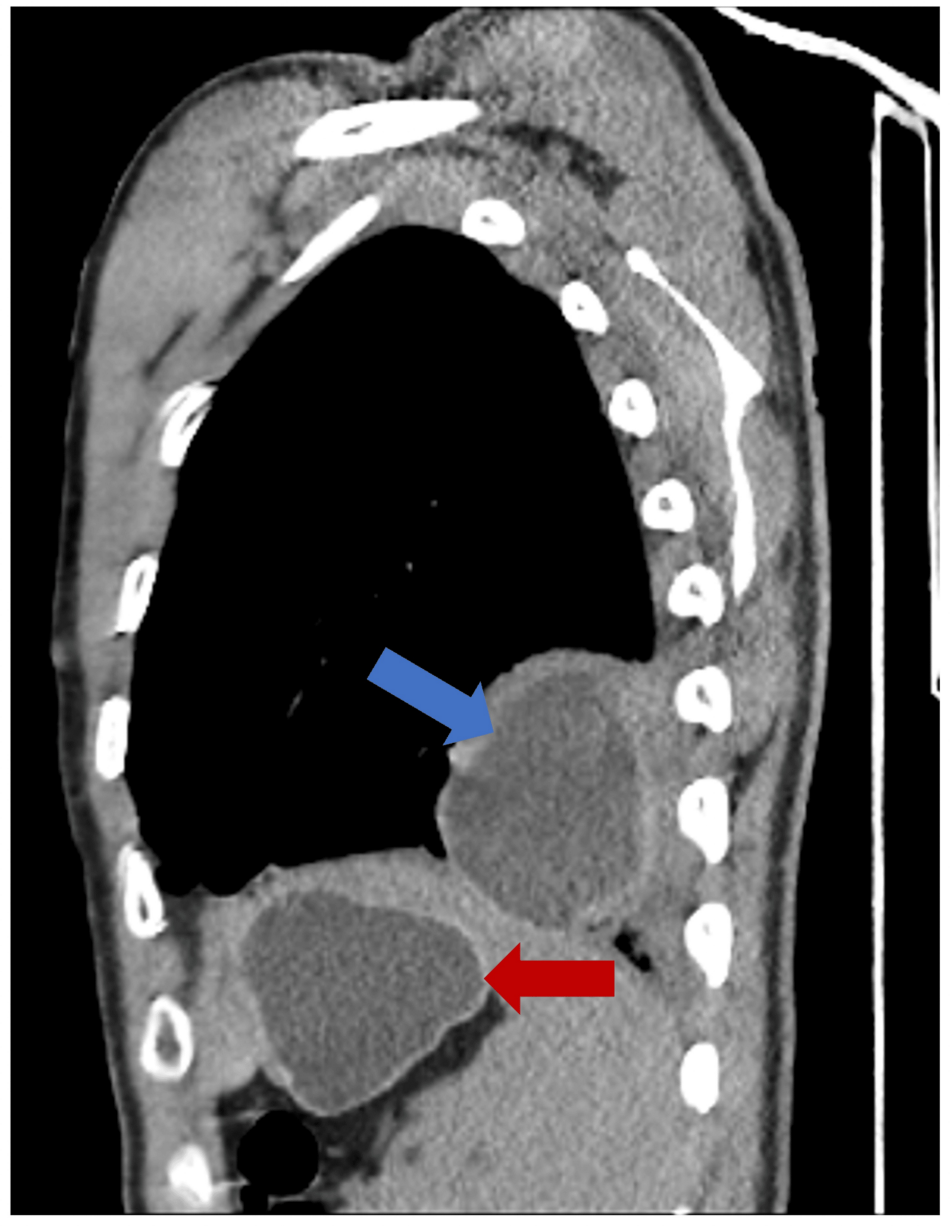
Thoracic and abdominal CT scan (sagittal view) revealing two hydatid cysts. The blue arrow shows a hydatid cyst in the lower lobe of the left lung; the red arrow shows a hydatid cyst in the left liver lobe. CT, computed tomography
